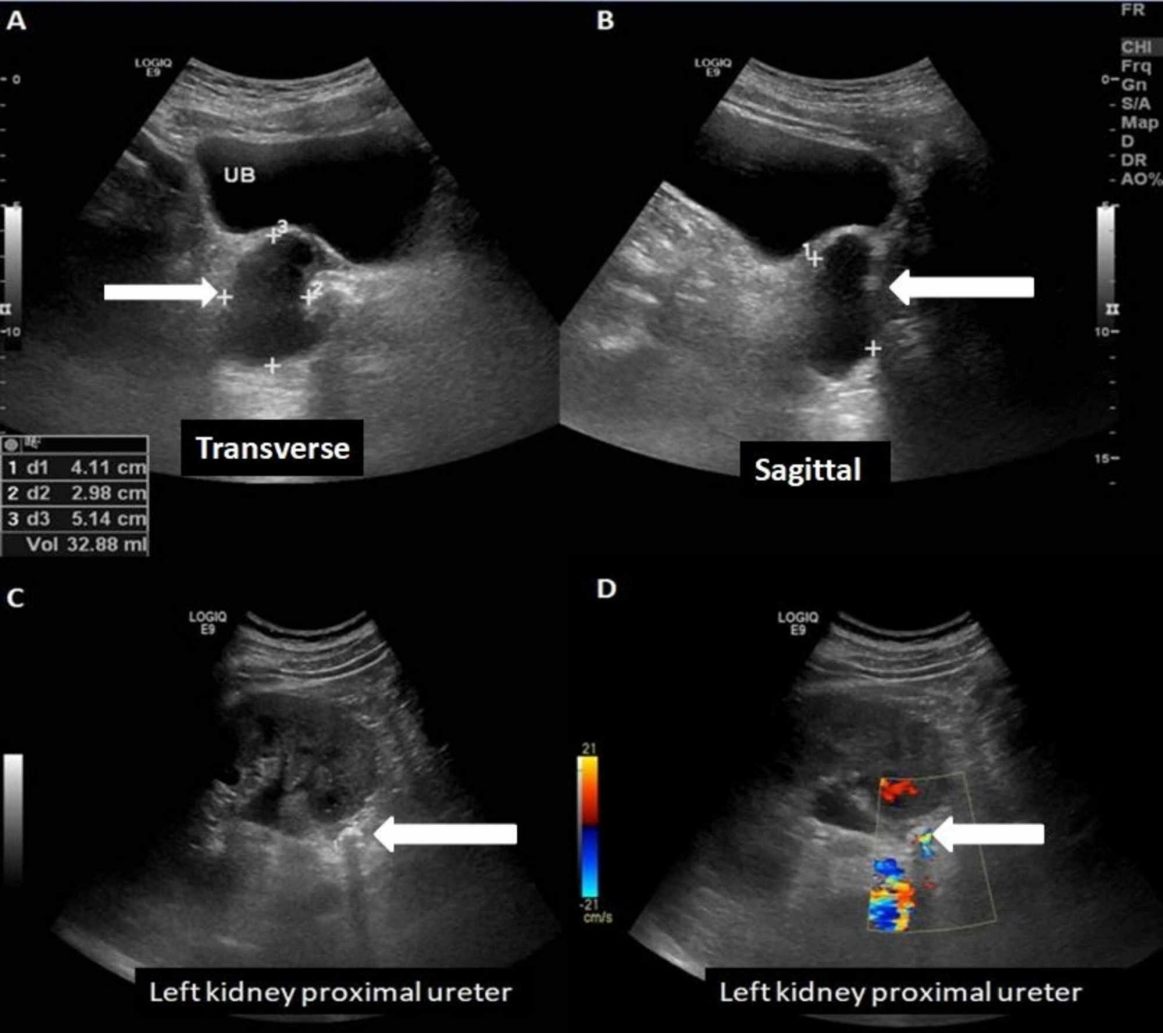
Ultrasound of the abdomen and pelvis A well-defined cystic lesion with faint internal echoes/debris, which is indenting the right posterolateral urinary bladder wall (A, B). A hyperechoic structure with posterior acoustic shadowing and twinkling artifact in the proximal ureter, associated with upstream pelvicalyceal dilatation, consistent with an obstructing stone (C, D).
